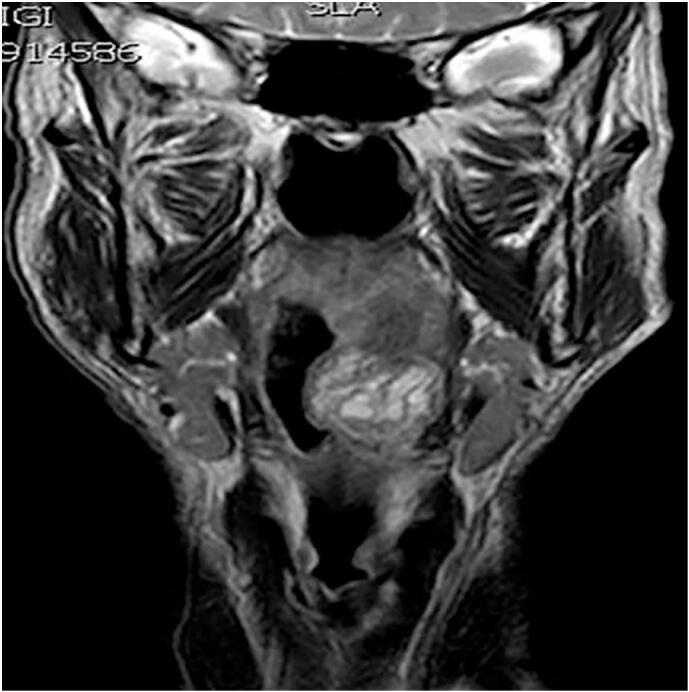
High intensity with hypointense areas at T2 MRI: coronal scan.
